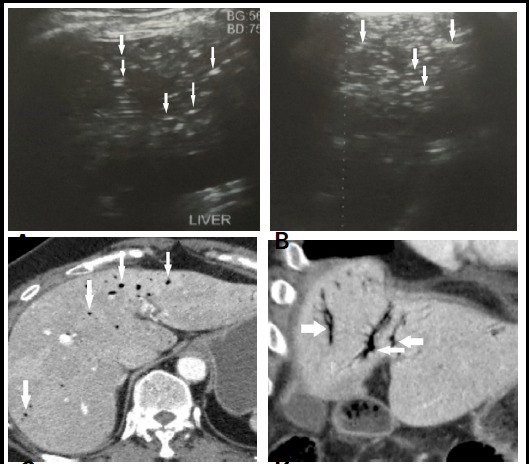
A), B) Ultrasound images showing air in intrahepatic portal vein branches. C) CECT images showing air in the intrahepatic portal vein branches.
